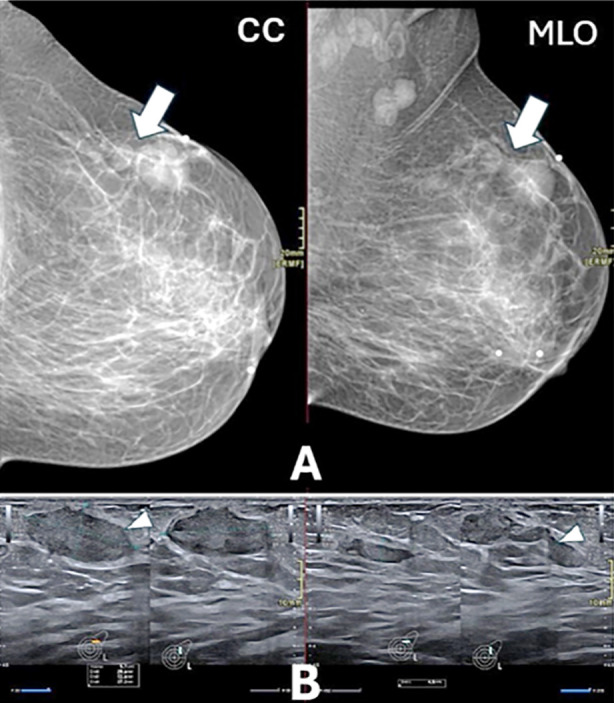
Radiographic images of an elderly female with a painful breast lump. (A) Left breast mammogram (CC and MLO views) showing two partly defined high density nodules (arrows) in the upper outer quadrant of left breast underlying the site of metallic marker and (B) Ultrasound of the same patient shows intercommunicating pockets of collection with internal homogenous avascular echoes.
